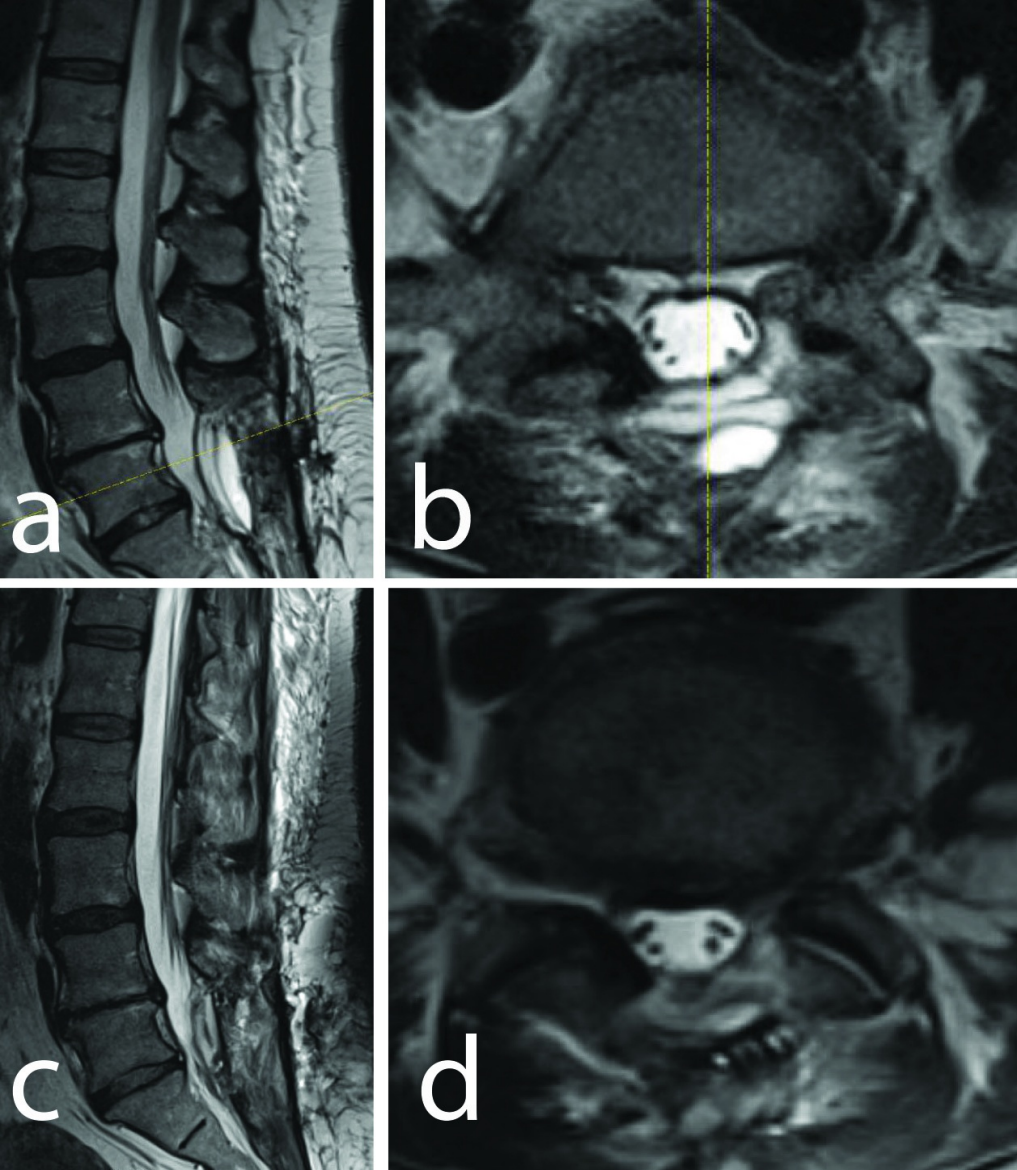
Axial and Sagittal T2W Images (a) Sagittal T2-weighted magnetic resonance imaging (MRI). (b) Axial T2-weighted MRI at the level of L4-5 taken postoperatively demonstrating decompression of the entire SSEH following L5 laminectomy with intradural exploration and arachnoid fenestration. (c) Sagittal T2-weighted MRI (d) Axial T2-weighted MRI at the level of L4-5 taken one month postoperatively demonstrating continued resolution of the entire SSEH.
